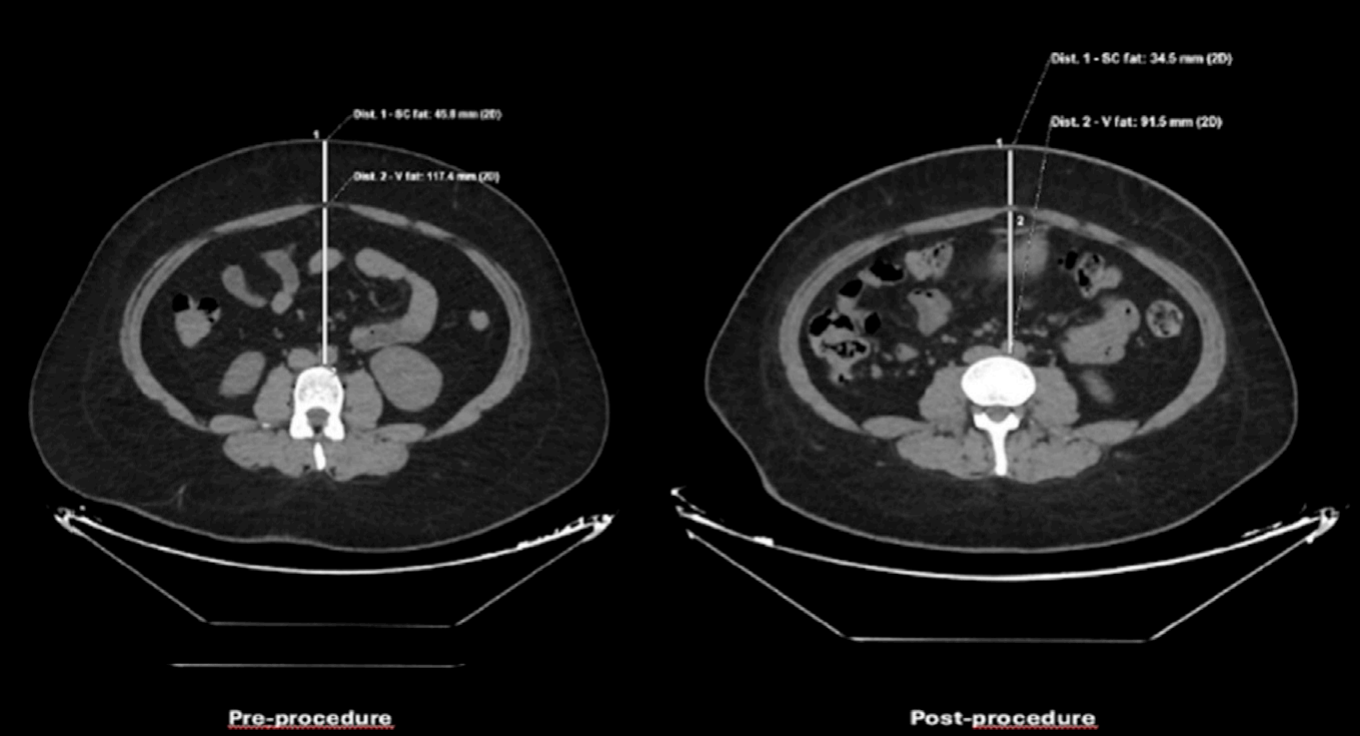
Pre-procedure and post-procedure measurements of visceral fat and subcutaneous fat tissue thickness on a patient’s CT scan.
